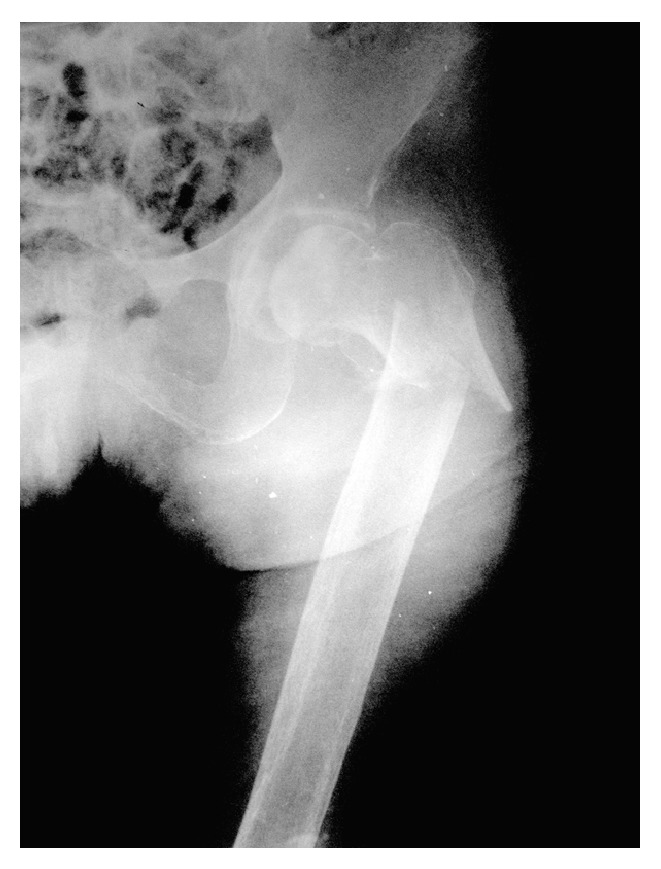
Left intertrochanteric hip fracture seen on radiography. It was understood from the widened femoral canal that osteoporosis was associated with the fracture.
